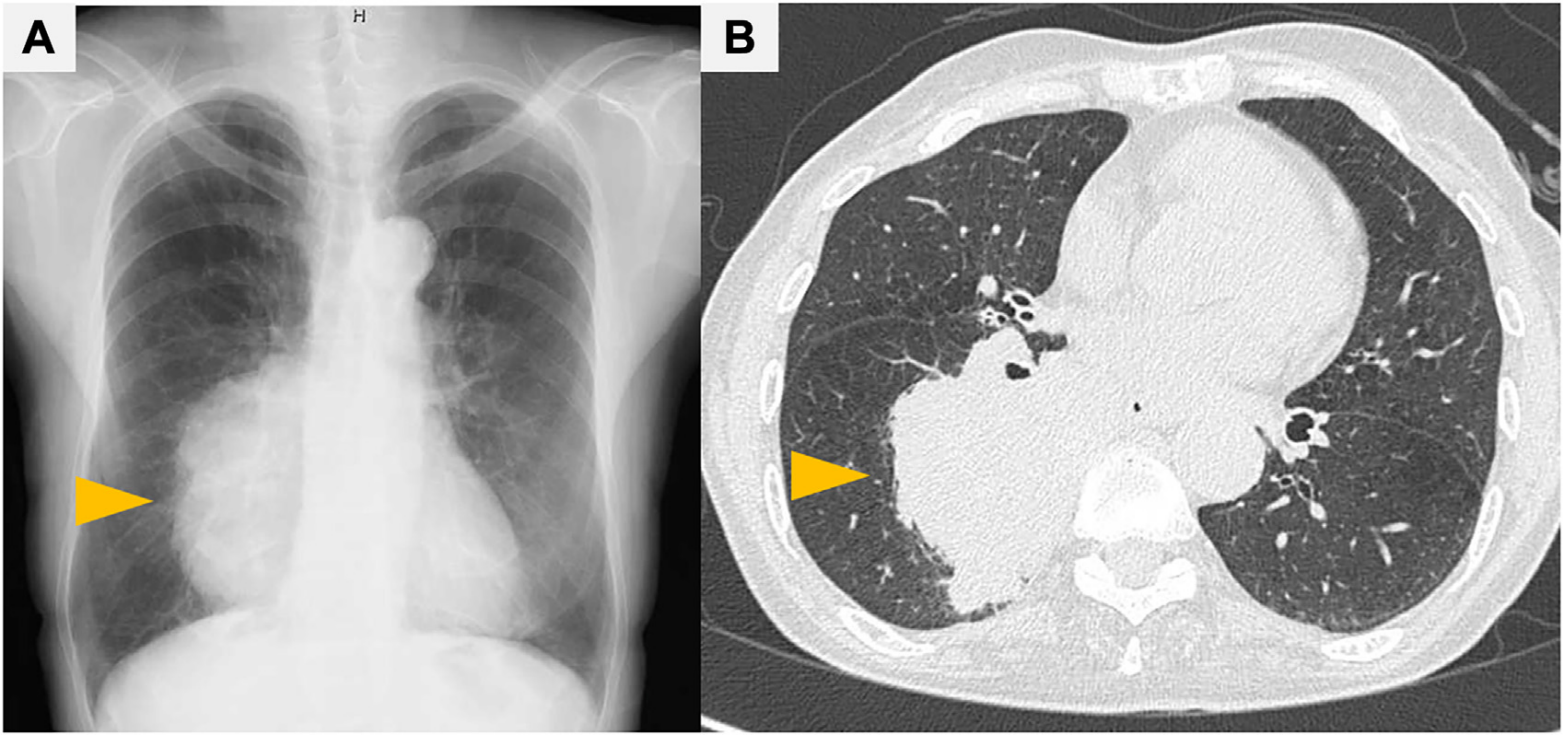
Perioperative imaging. (A) Chest radiography revealing a huge lung tumor in the right lung field (arrowhead). (B) Computed tomography showing a >10-cm tumor in the right lower lobe (arrowhead).
